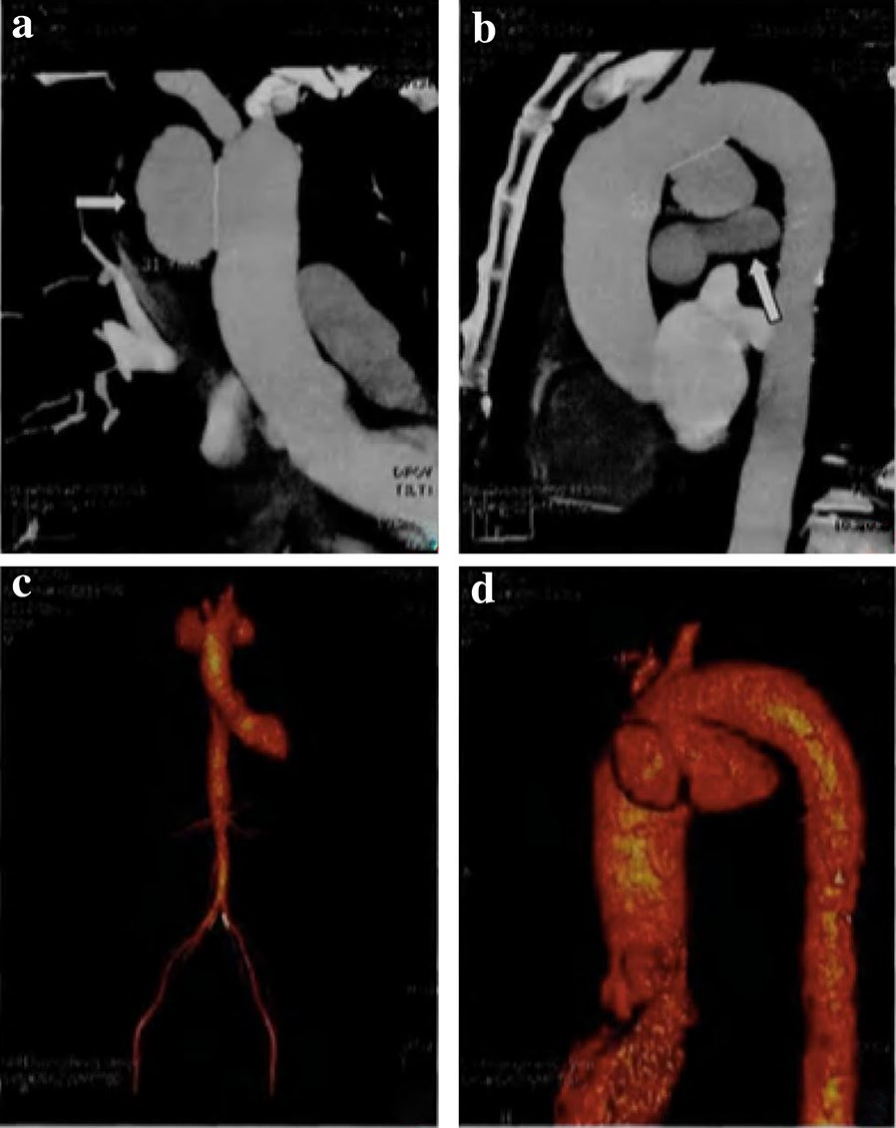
CTA and 3D reconstruction of the whole aorta. a and b showed multiple tumor-like bulges in the dilated ascending aorta and the arch of aorta with calcification lesions in the aortic wall. The maximum cross-sectional diameters of the dilated segments were 49.1, 33.9 and 19.2 mm. c and d showed the 3D reconstruction imaging of whole aorta before treatment
